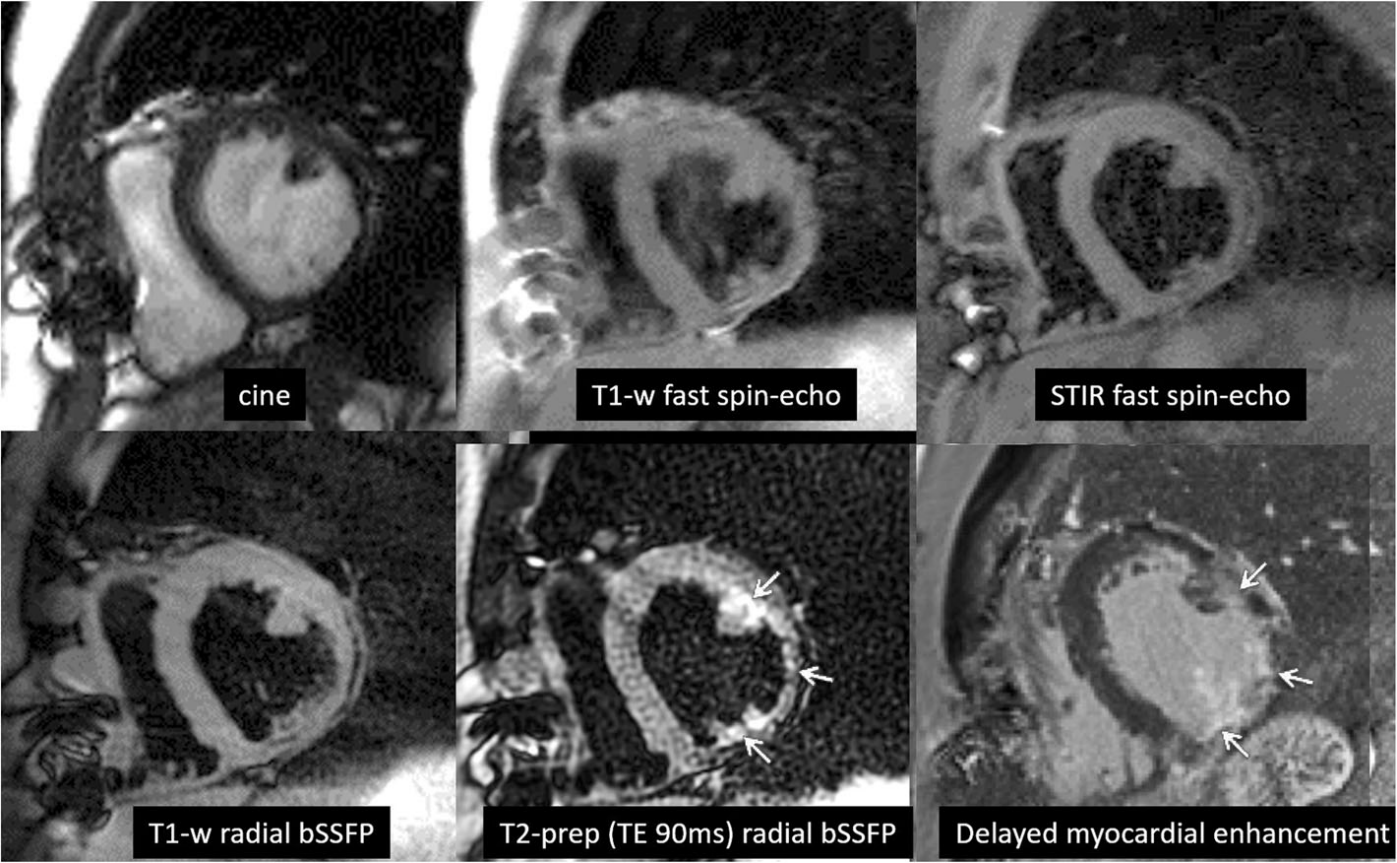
Patient with ischemic cardiomyopathy and history of coronary artery bypass grafts. Top row from *left* to *right*: systolic frame from cine bSSFP, T1-weighted fast spin-echo, T2-weighted STIR fast spin-echo. Bottom row from left to right: T1-weighted radial bSSFP, T2-weighted (T2_prep_ time = 90 ms) radial bSSFP, delayed myocardial enhancement using inversion recovery spoiled gradient-echo. The cine image shows inferolateral left ventricular wall thinning. The T1-weighted and T2-weighted fast spin-echo images show wall thinning but are otherwise unremarkable. In comparison with fast spin-echo, T1-weighted radial bSSFP appears less blurred. T2-weighted radial bSSFP shows a distinct subendocardial T2-signal abnormality corresponding to the region of delayed enhancement, which was not prospectively identified in the fast spin-echo images. (Low signal regions in the anterior chest wall are caused by sternal wires)
